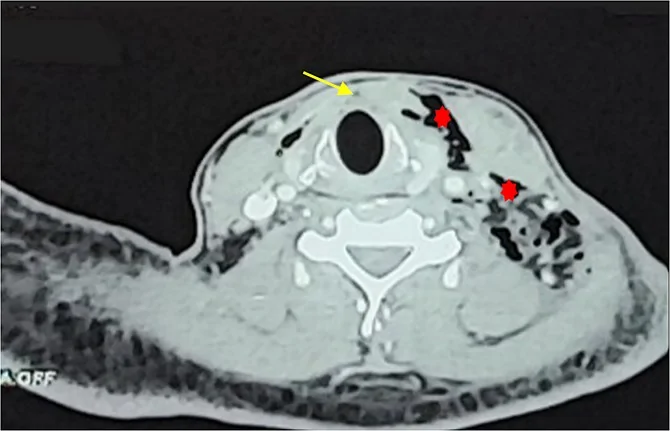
Axial contrast-enhanced cervical CT scans showing subhyoid fluid collections (yellow arrows) adjacent to the thyroid cartilage, associated with emphysema (red asterisks), consistent with cervicothoracic cellulitis.
